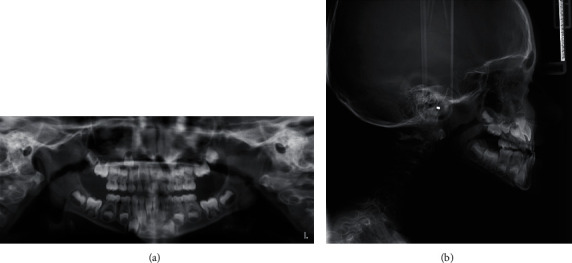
Pre-treatment radiographic records: (a) orthopantomogram and (b) lateral cephalogram.
